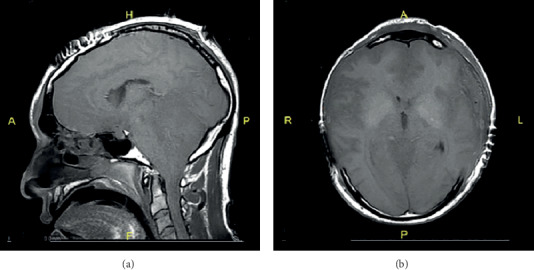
MRI post 72 hours of admission. After uncomplicated wide bilateral craniectomies. (a) The sagittal view showing transtentorial herniation with stretching and obliteration of the fourth ventricle. (b) Note on the axial view showing diffuse swelling with loss of gray white junctions and absence of ventricular space.
